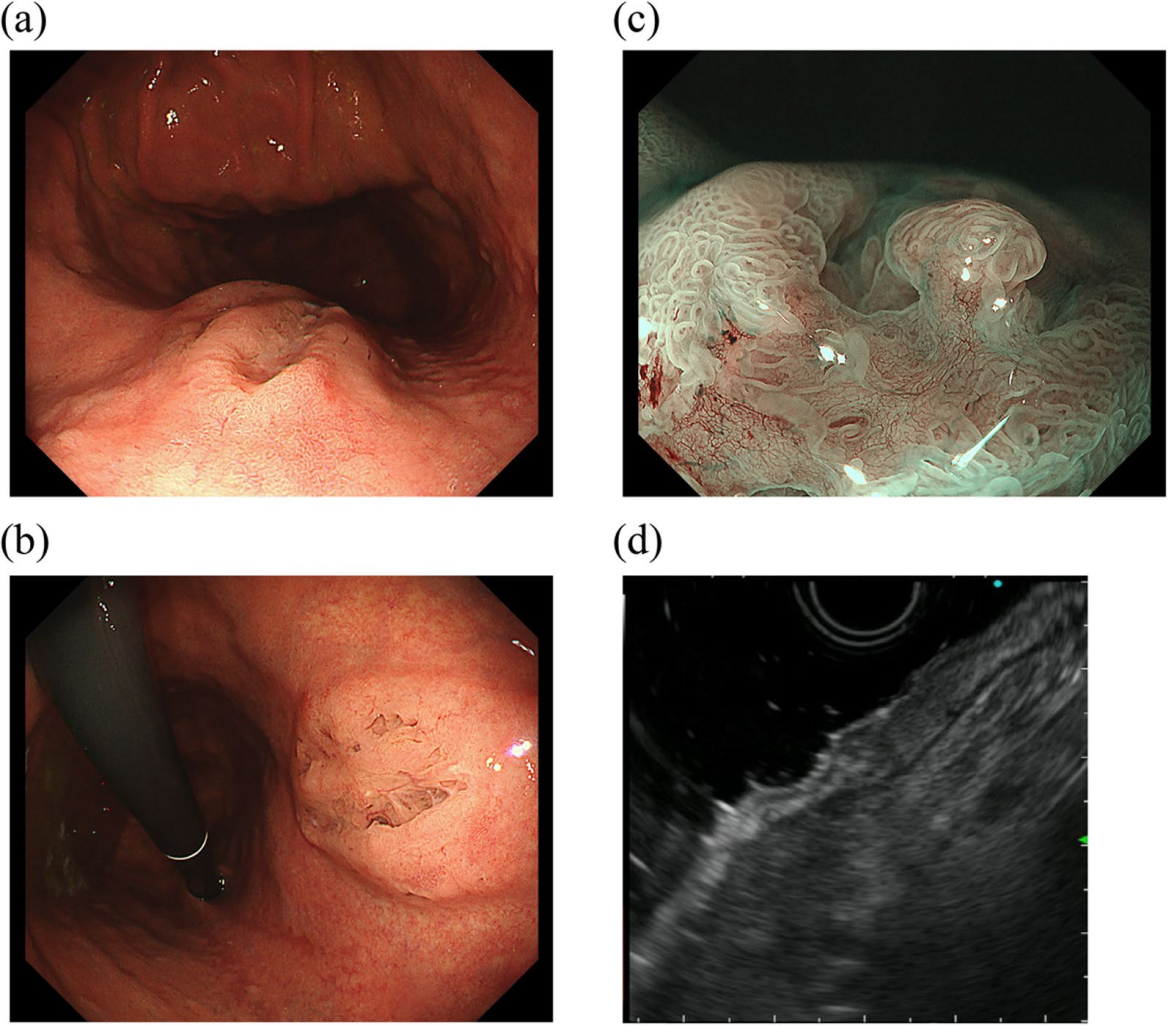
0–IIa + IIc lesion measuring 30 mm in size with a depressed surface at the lesser curvature side of the middle of the gastric body (a and b). Magnifying NBI revealed the vascular structure at the center of the lesion (c). Endoscopic ultrasonography revealed an isoechoic tumor with an elongated anechoic area suggestive of an ectopic pancreas, and also showed that the deep third layer and forth layer were remained (d)
